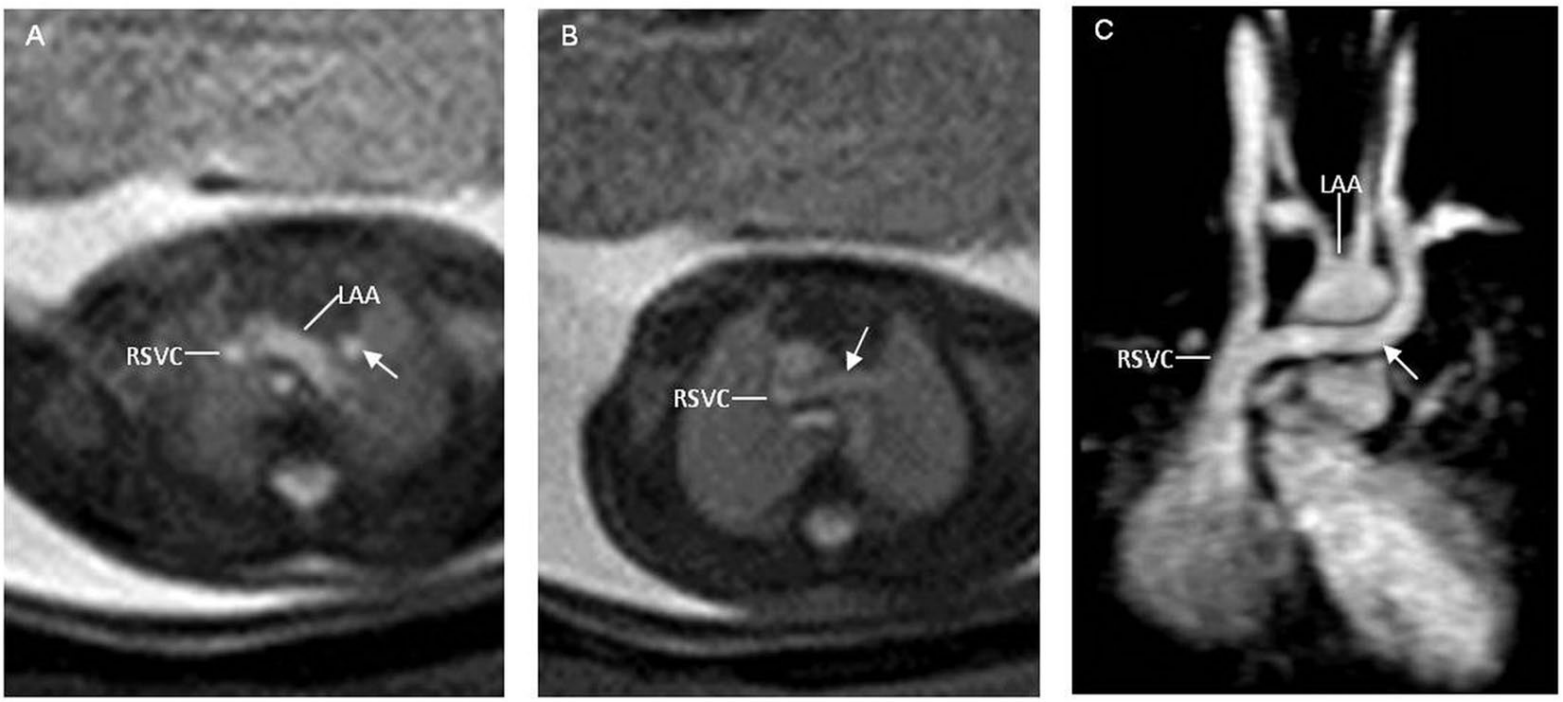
A 25-week fetus with an abnormal subaortic left brachiocephalic vein (ASLBV) with a left aortic arch. Fetal CMR B-TFE transverse view images show an abnormal subaortic left brachiocephalic vein (arrows in A and B) courses below the left aortic arch (LAA in A) into right superior vena cava (RSVC in A and B) and a left aortic arch (LAA in A); Postnatal MRI image also shows the left brachiocephalic vein (arrow in C) courses below the left aortic arch (LAA in C) into right superior vena cava (RSVC in C).
